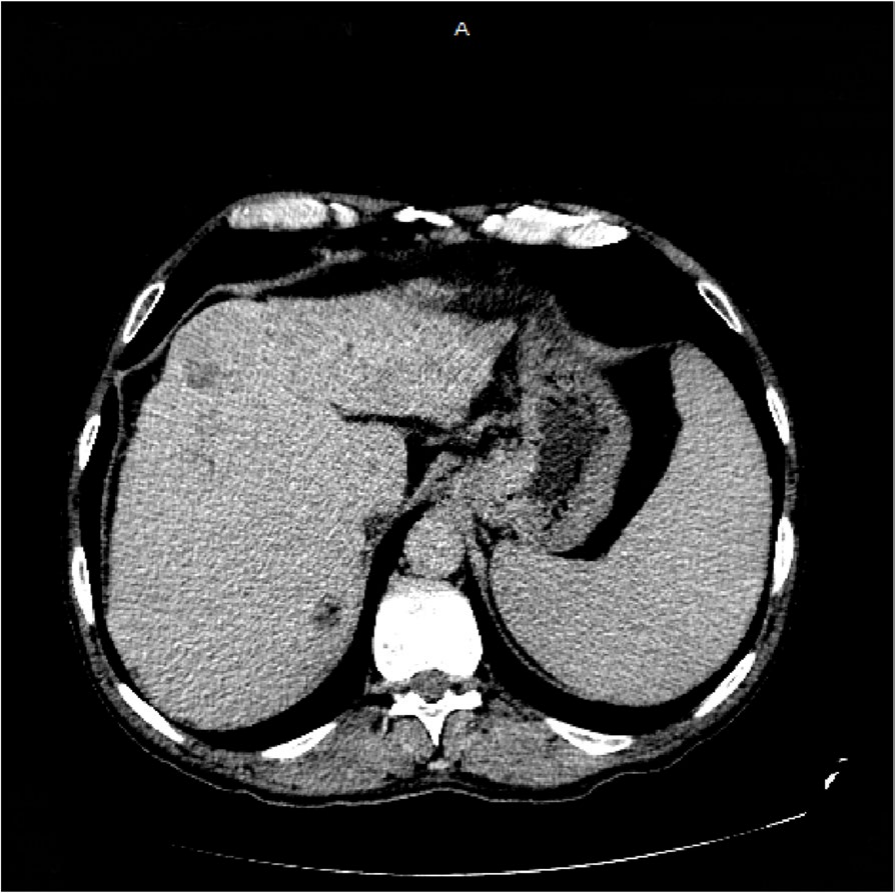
Delayed phase CT abdominal scan showing delayed washout, hypo vascular hepatocellular carcinoma
